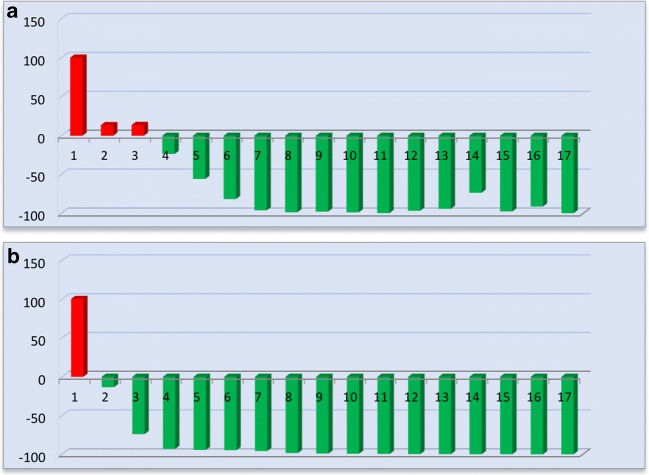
a Waterfall plot showing PSA response at 8 weeks after one cycle of ^225^Ac-PSMA-617 radioligand therapy. b Waterfall plot showing best PSA response to ^225^Ac-PSMA-617 radioligand therapy
